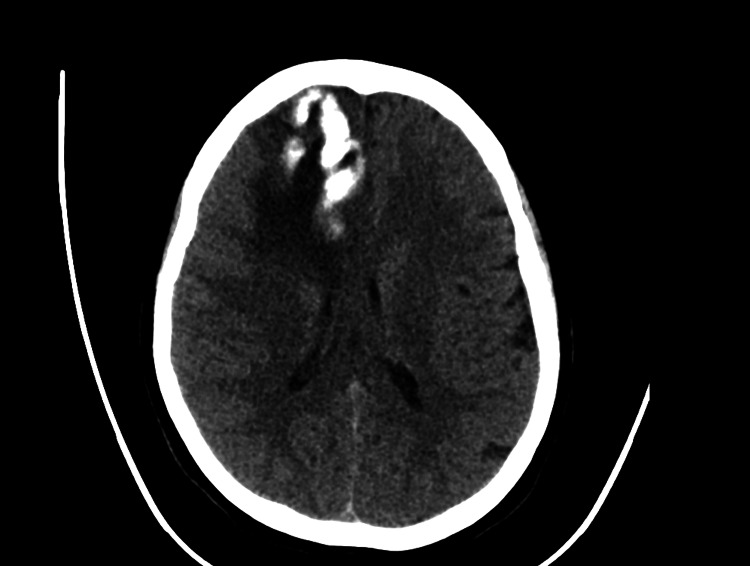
Brain CT showing hypodense right frontal subcortical lesion with calcification, vasogenic edema, and mass effect on ipsilateral frontal horn of the lateral ventricle.
